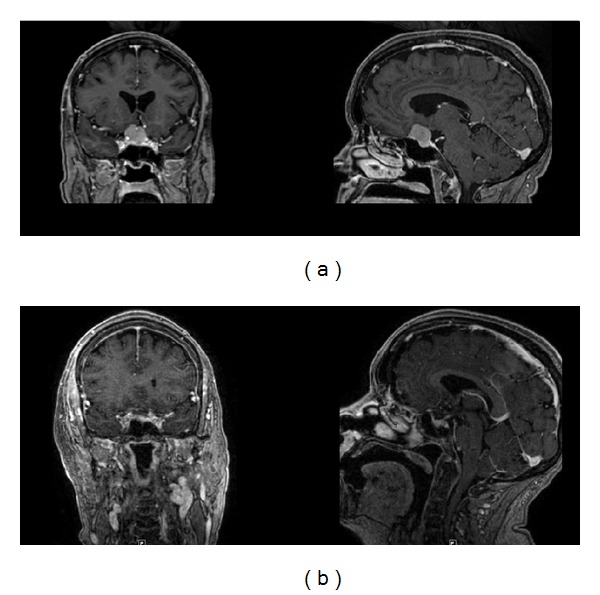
(a) Preoperative and (b) postoperative MR images of a homogeneously enhancing mass involving the tuberculum sellae and planum sphenoidale. Pathology was meningioma. Gross total resection was achieved.
